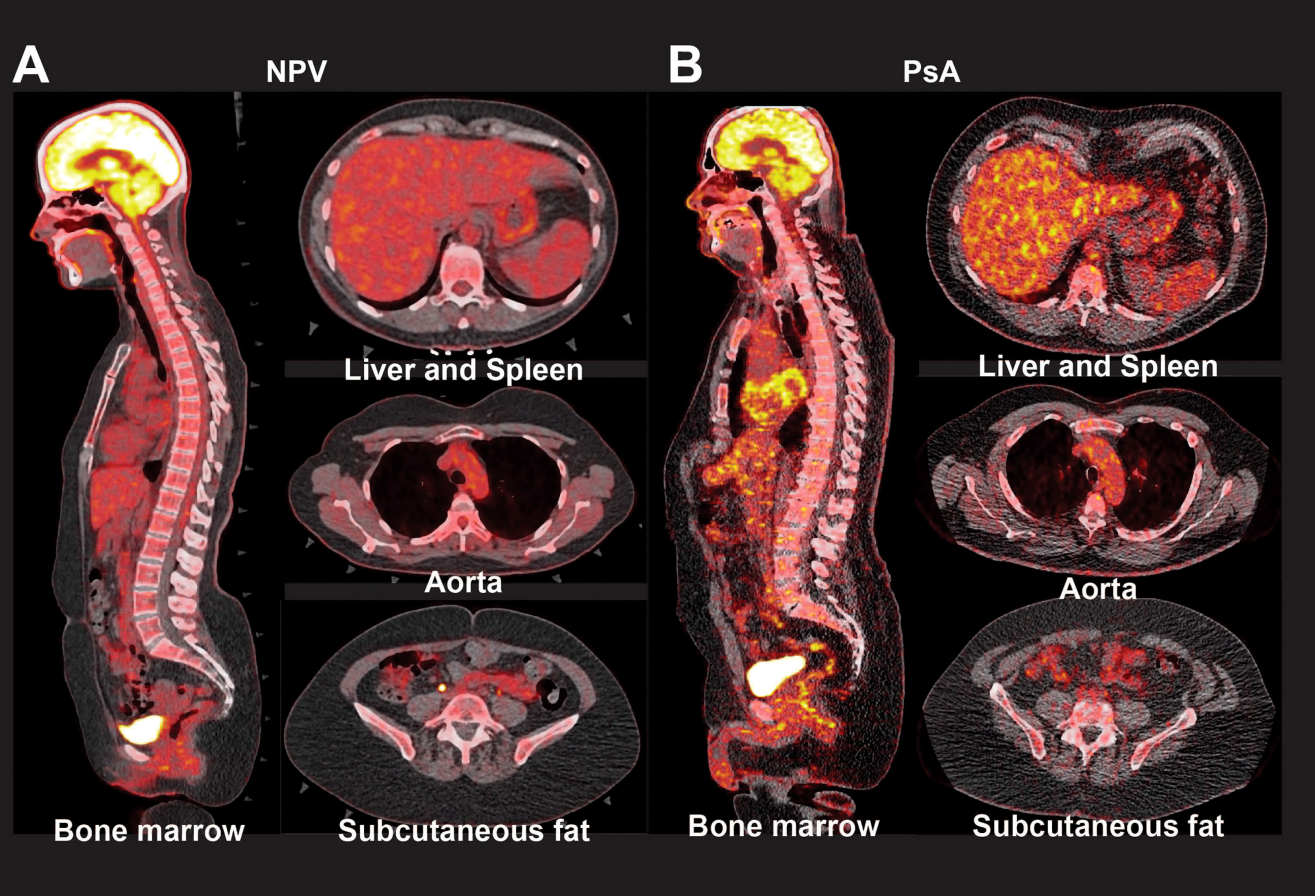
Radiographic markers of inflammation in patients with psoriatic arthritis. Representative PET-CT images show FDG uptake in the bone marrow, liver, spleen, aorta, and subcutaneous fat of non-psoriatic volunteers (NPV, A), and patients with psoriatic arthritis (PsA, B).
